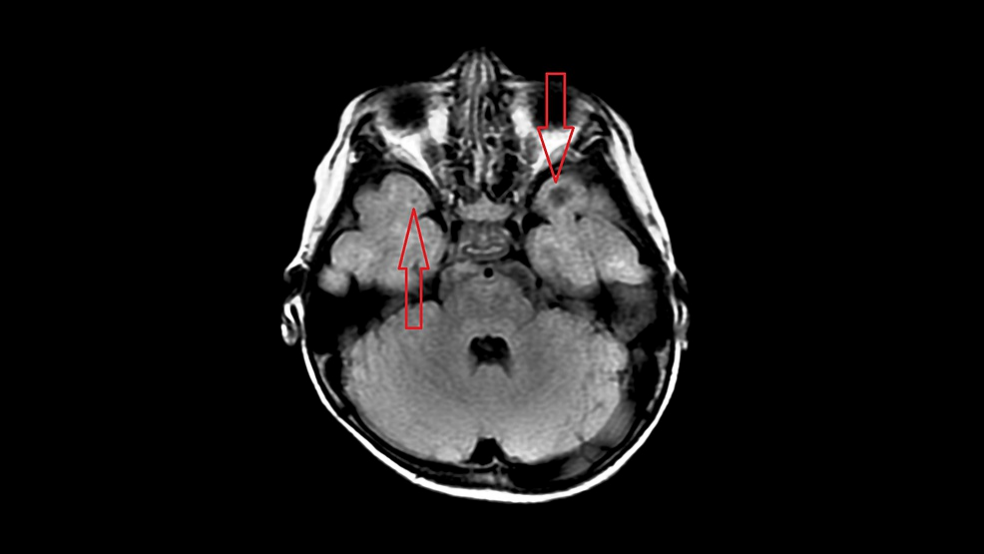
Axial T2 FLAIR image corresponding to T2W image of Figure 4 showing inversion of cystic fluid. FLAIR: fluid-attenuated inversion recovery.
